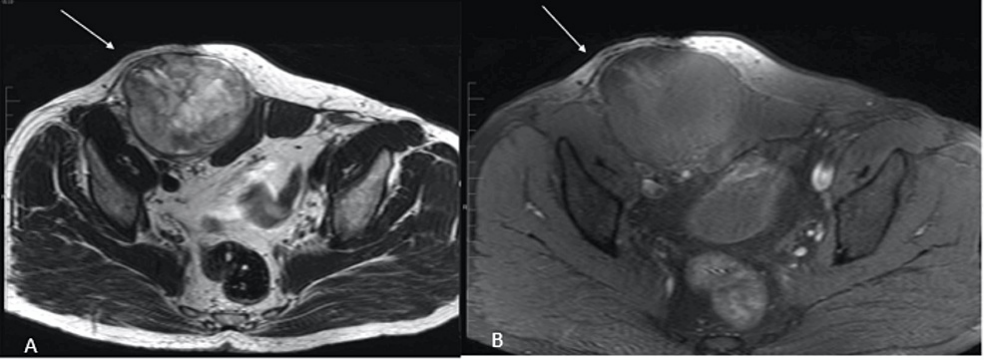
Axial T2-weighted images (A) and STIR (B) magnetic resonance imaging of the pelvis showing a right inguinal lesion (white arrows) STIR: short-TI inversion recovery
